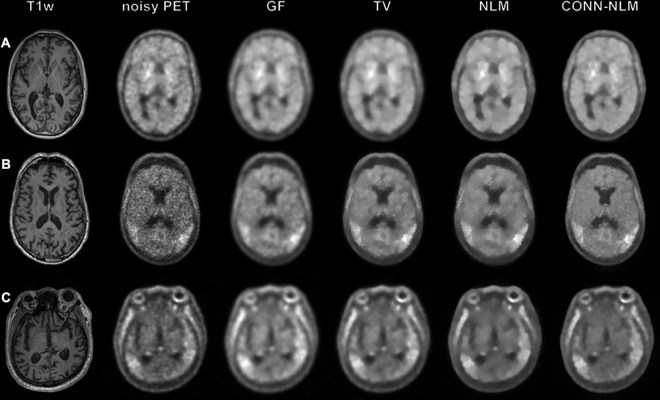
Applying image smoothing on clinical tau-PET and MRI data from (A) a health-control subject (B) a mild cognitive impairment patient and (C) a Alzheimer’s disease patient. From left to right: T1-weighted image, noisy PET image, Gaussian filtering (GF), total variation (TV), nonlocal means (NLM) and the proposed CONN-NLM filtering. Filtering parameters were chosen for all filters to produce approximately the same level of image noise.
